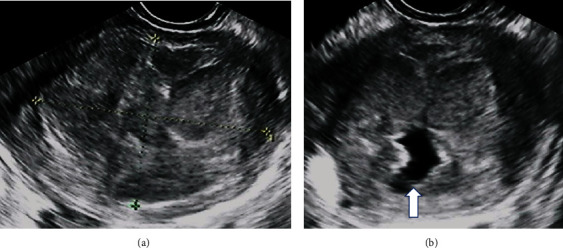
Transvaginal ultrasound images: (a) the tumor margin and the underlying myometrium were hyperechoic and well defined. Several small nodules within a large mass were isoechoic and hypoechoic compared to the normal uterine myometrium; (b) an anechoic unilocular cystic area within a small nodule was observed (indicated by the arrow).
